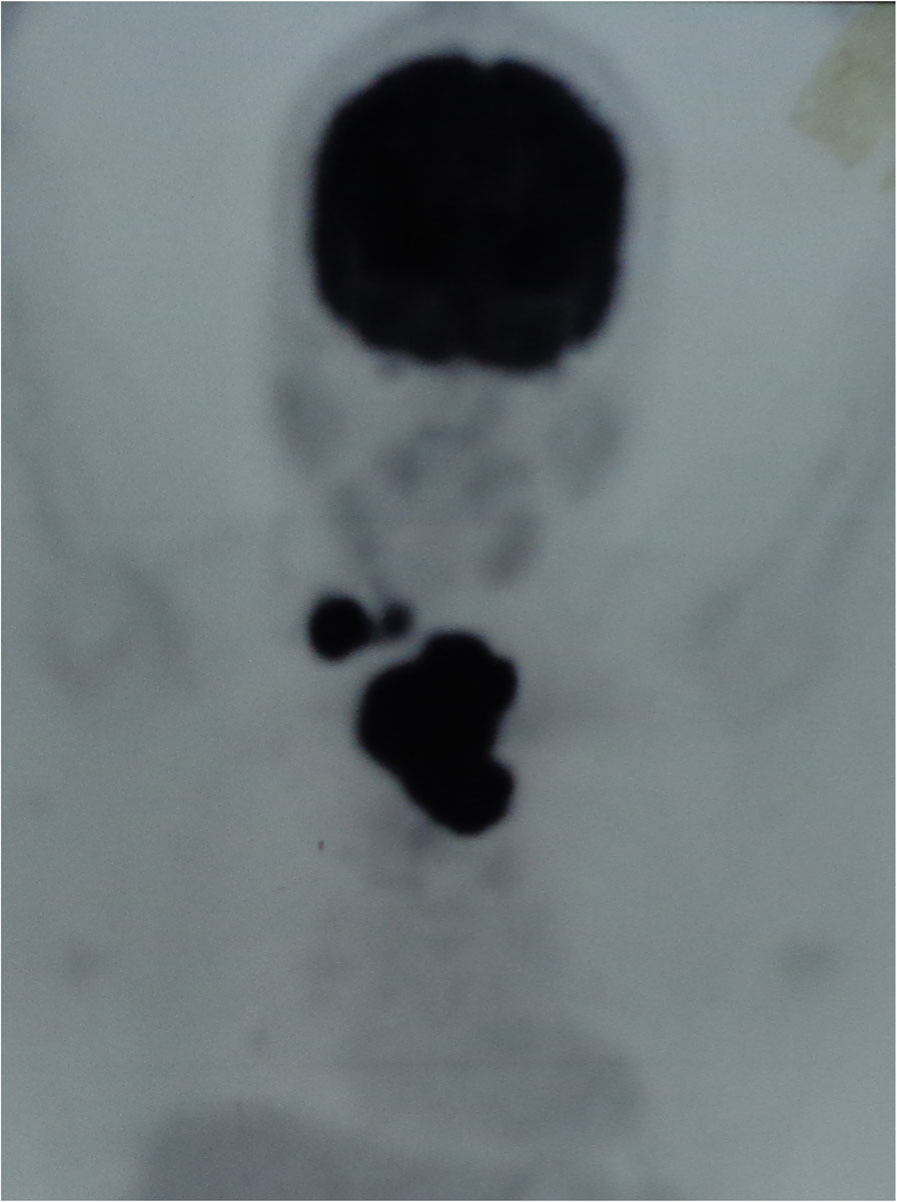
PET CT showing FDG uptake in the mass and neck node
